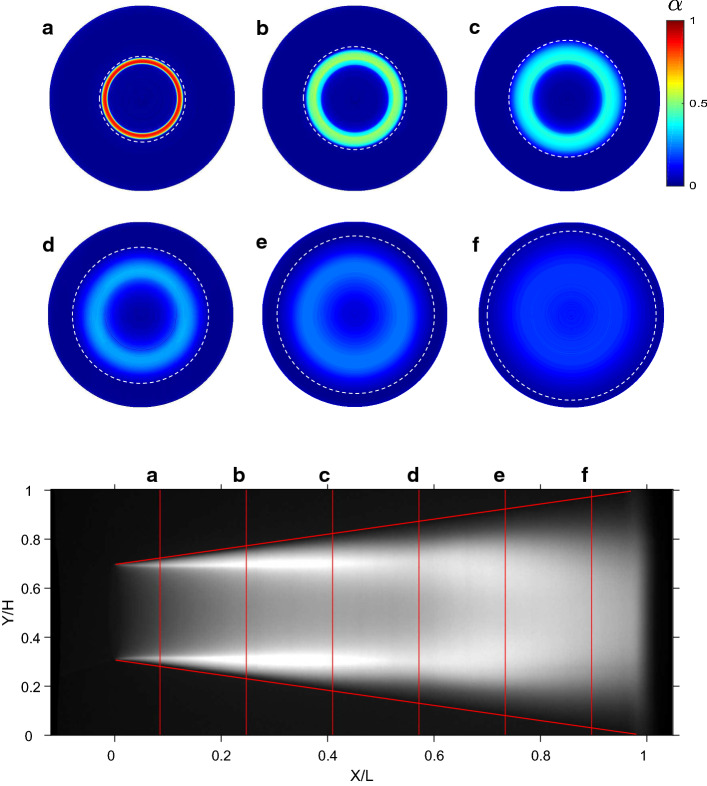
Void fraction in a cavitating venturi, obtained using X-ray computed tomography. The cross sections a–f are taken at various downstream positions. Reproduced from Jahangir et al. [138] with permission ©2019 Elsevier
